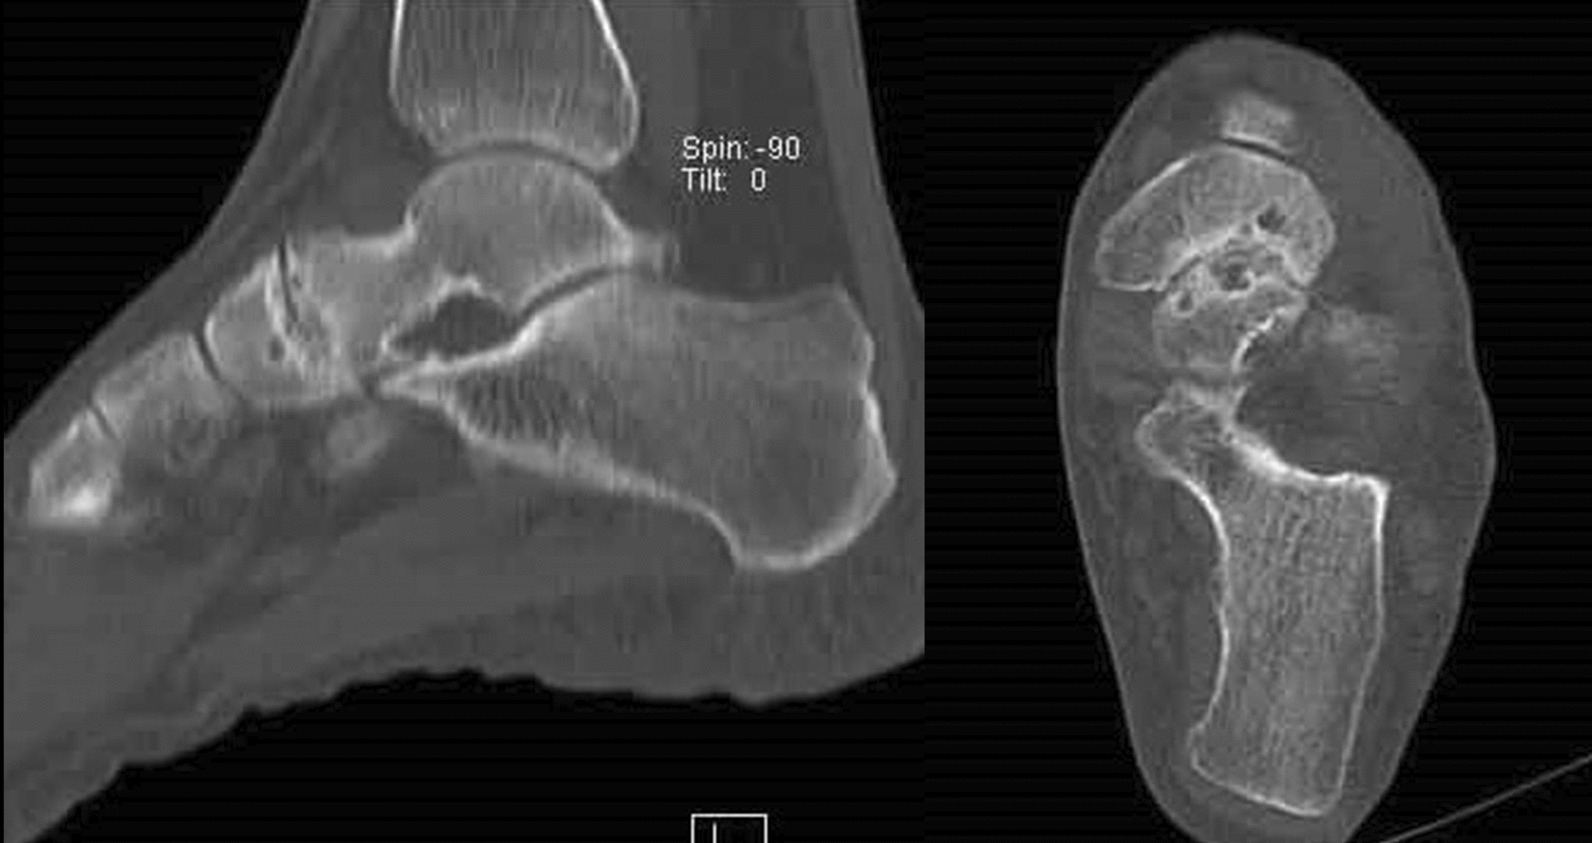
Sagittal and coronal CT scans showing cortical erosion and sclerosis, joint space narrowing and subchondral bone cysts in the talonavicular joint
